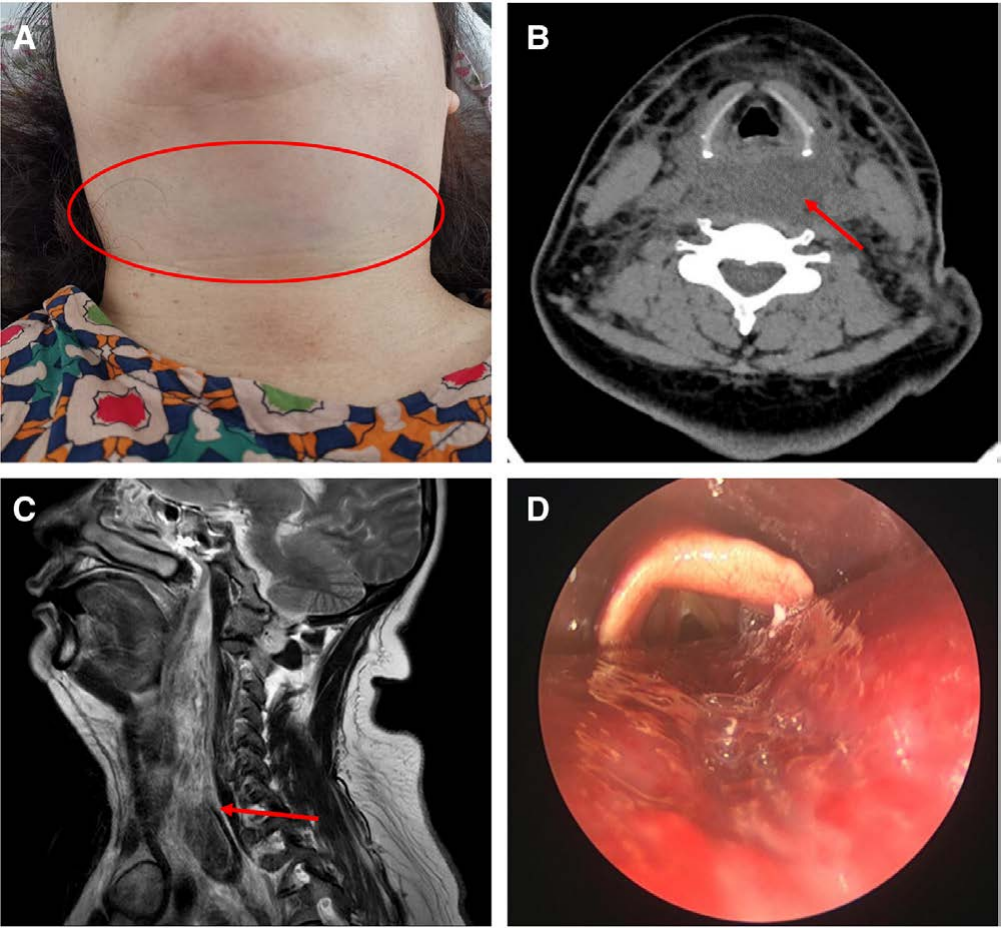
The patient’s physical signs and imaging findings before treatment. (A). Bruised spot on patient’s neck (roundel). (B). Axial CT shows hematoma (arrow) at the level of the arytenoid cartilage. (C). Sagittal MRI shows the extent of the hematoma (arrow). (D). Laryngoscopy shows that the posterior wall of the hypopharynx is bulged, the mucosa shows a dark red color, and it obstructs the posterior three-fourths of the glottic region area. CT = computed tomography, MRI = magnetic resonance imaging.
